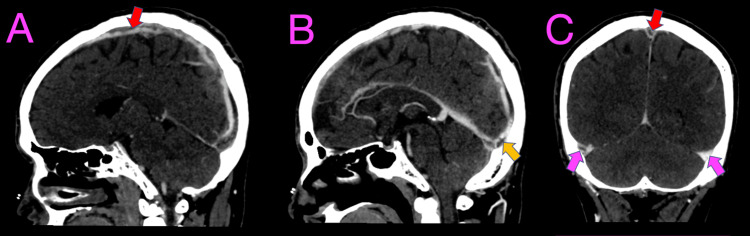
Computed tomography venogram of the brain. Computerized tomography venogram of the brain showing extensive thrombosis as filling defect in the superior sagittal sinus (A, C) (red arrows) with extension to straight sinus (B) (yellow arrow), and bilateral transverse sinuses (C) (pink arrows).
